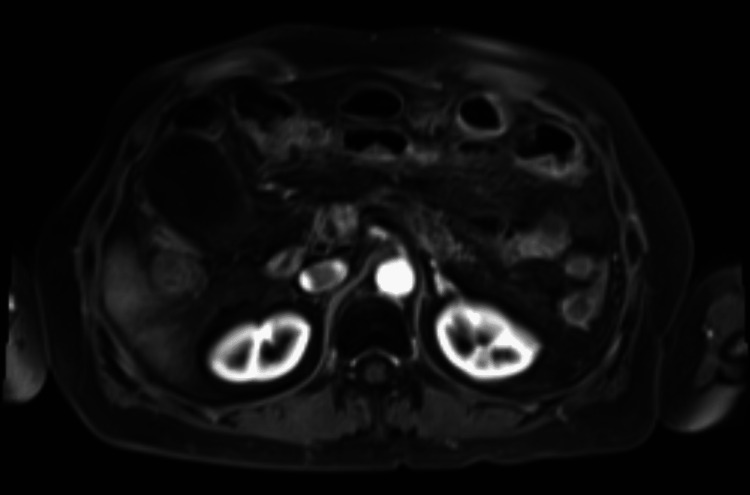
MRI, axial plane: small amount of free peritoneal fluid
